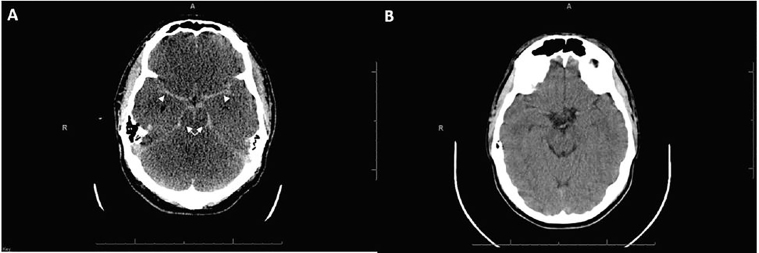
A) Post-resuscitation axial computed tomography (CT) of the brain demonstrating diffusely increased density of the basal cisterns (arrows) and subarachnoid spaces (arrowheads); and B) normal axial CT of the brain obtained on prior visit.
